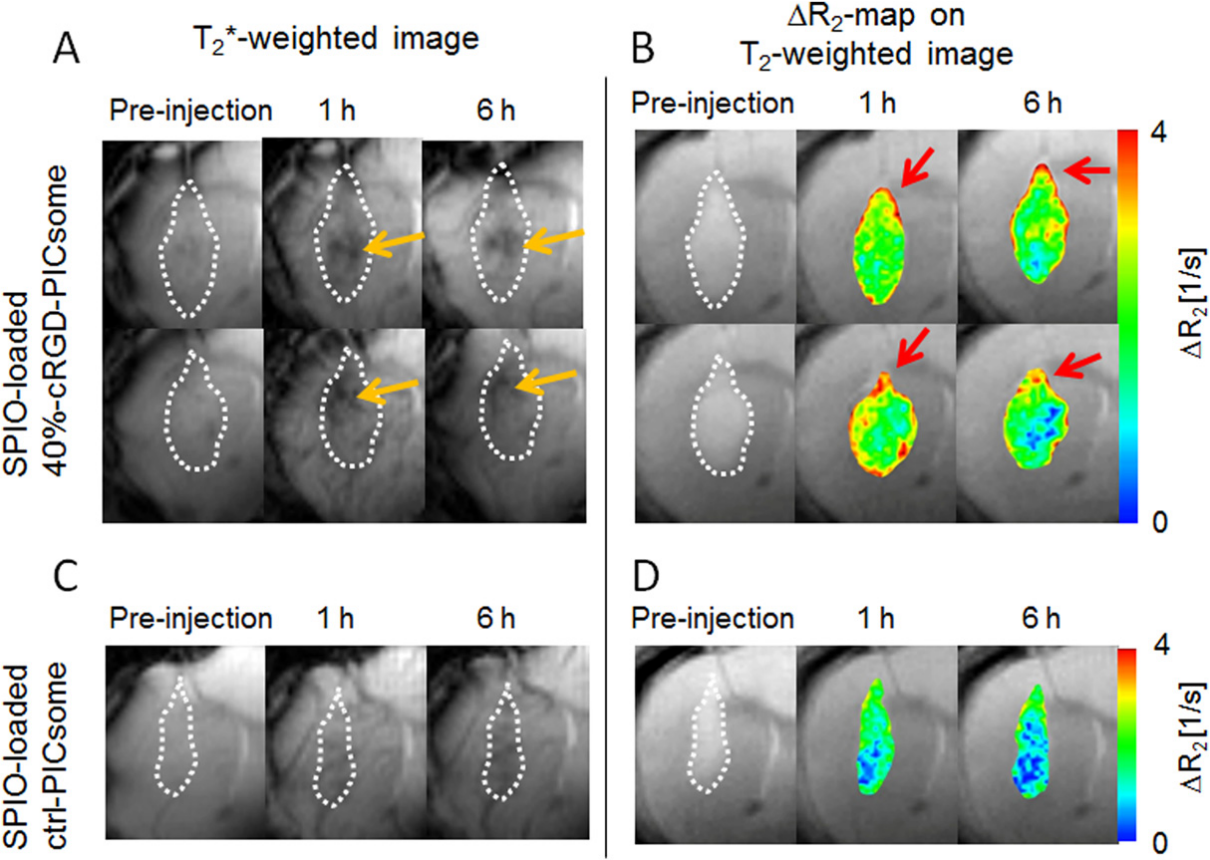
MRI of U87MG orthotopic glioblastoma with SPIO-loaded PICsomes. *T*_2_∗-weighted MR images of (A) 40%-cRGD-PICsomes (*n* = 2) and (C) Ctrl-PICsomes (*n* = 2) before, and 1 and 6 h after administration. (B) *ΔR*_2_ maps in the tumor 1 and 6 h after PICsome administration compared with pre-administration. Tumor lesions were identified using *T*_2_-weighted MRI, and are circled with white dashed lines. Orange arrows represent a reduction of the signal of the tumors. Red arrows represent the sites with significantly increased *ΔR*_2_ values.
